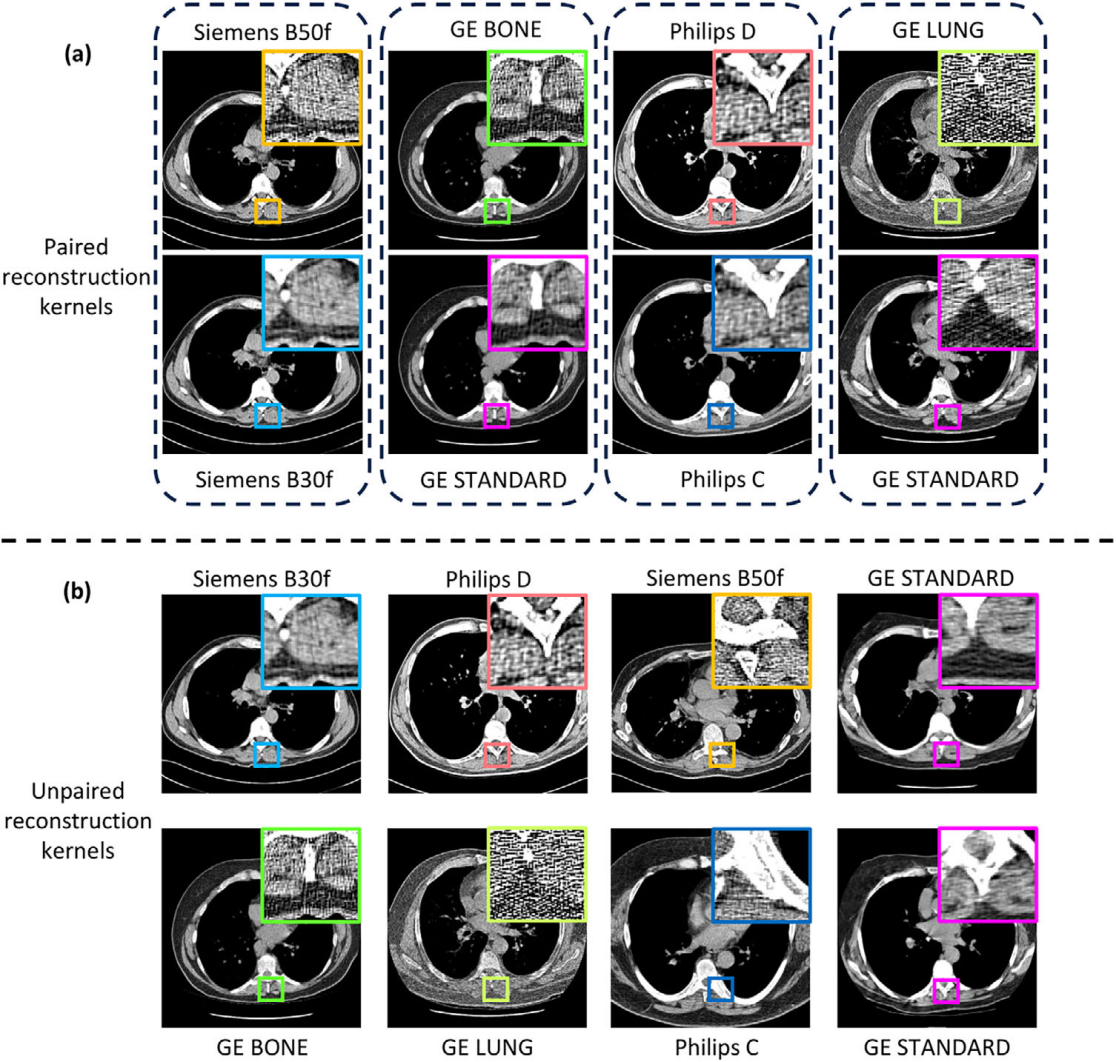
Reconstruction kernels influence the noise and resolution of the underlying anatomical structure in a computed tomography image. (a) Paired reconstruction kernels obtained from a given vendor exhibit a one‐to‐one pixel correspondence between the scans, which enables kernel harmonization. However, (b) across vendors, unpaired kernels show differences in anatomy, scan protocol, field of view, and reconstruction window. This creates additional difficulties that make harmonization a more challenging task.
